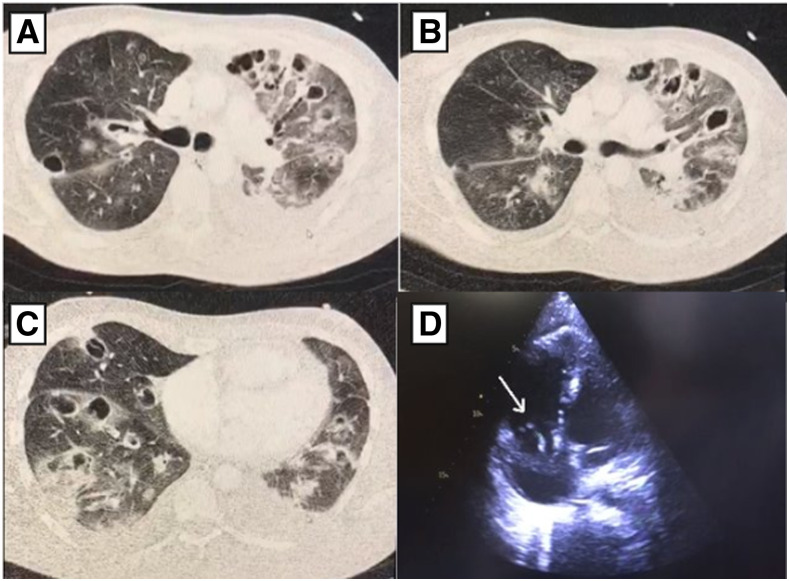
CT pulmonary arteriography. (A, B, and C) Multiple cavitating lesions throughout all lung fields, suggestive of septic pulmonary emboli associated with bilateral glass-ground opacities, and transthoracic echocardiogram. (D) Mobile vegetation on the tricuspid valve measuring 5 cm.
